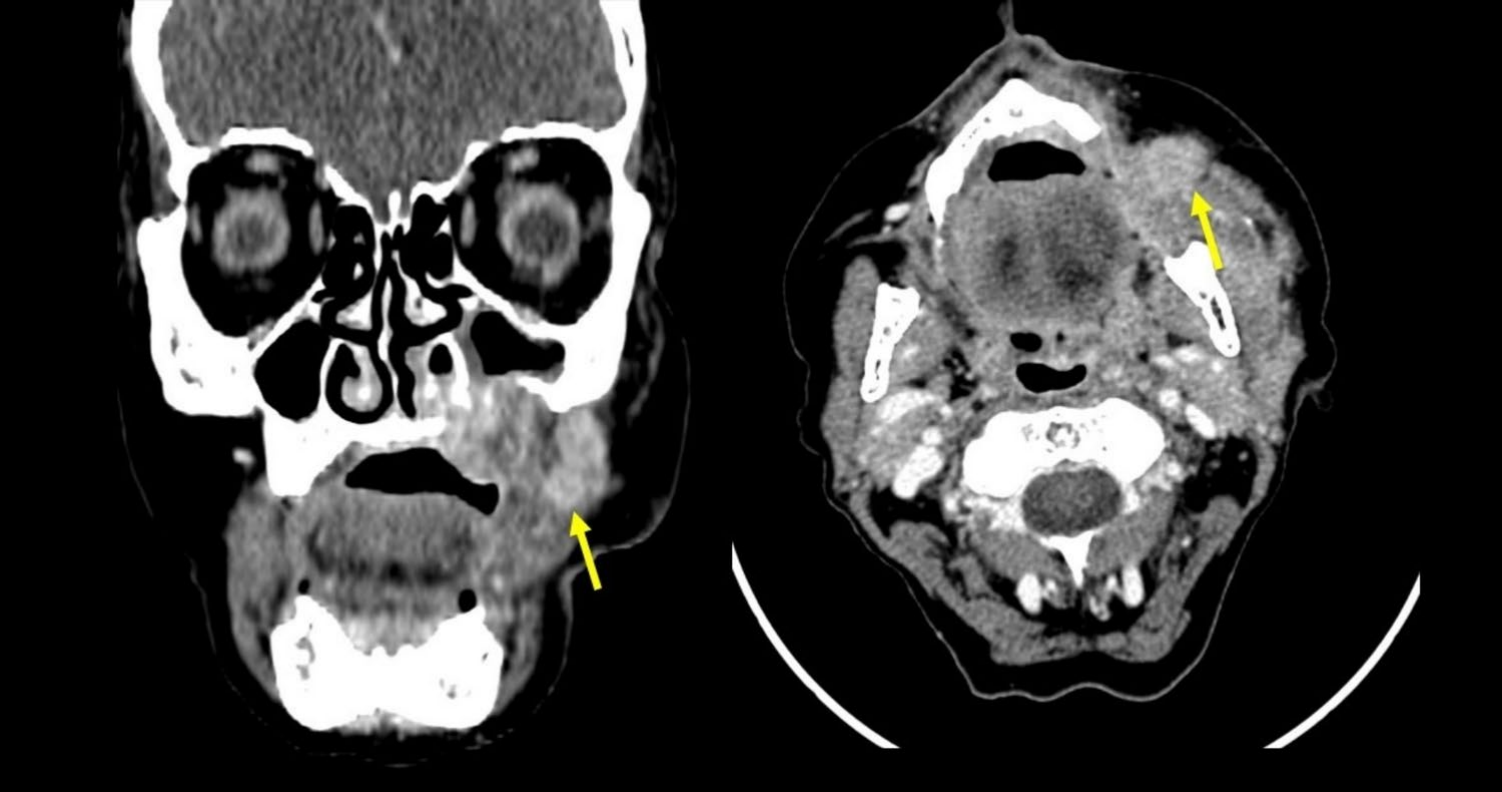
Coronal and axial views of post-operative CE-CT after 6 weeks in Patient with pT4aN1 superior left retromolar trigone SCC surgically treated with partial maxillectomy, neck dissection and temporal flap reconstruction. NI-RADS 3 for *T* because there is enhancing nodular area along the anterior margin of the flap (yellow arrow)
